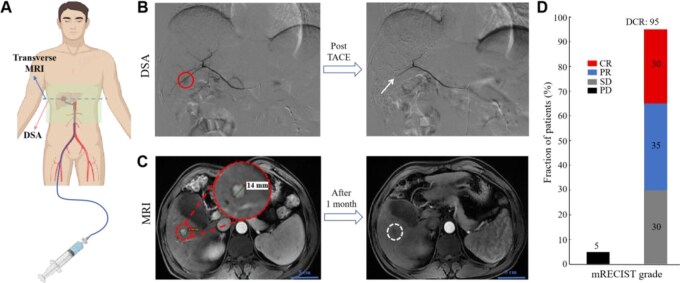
Clinical trial results. (A) Scheme of clinical case utilizing WizSphere® for TACE treatment of HCC. (B) a representative case of 51-year-old male: DSA image before and post-embolism. The red cycles show the lesion location. (C) Transverse MRI of the lesion before TACE treatment and 1-month follow-up MRI (baseline tumor diameter 14 mm, scale bar 5 cm). (D) Clinical cases statistical data at 3-month follow-up (20 cases). The *X*-axis indicates the tumor category after TACE treatment by mRECIST standard (Supplementary Table S5), and the *Y*-axis represents the fraction of each category relative to the total number of operation patients. CR, complete response; PR, partial response; SD, stable disease; PD, progressive disease; DCR, disease control rate (CR + PR + SD).
